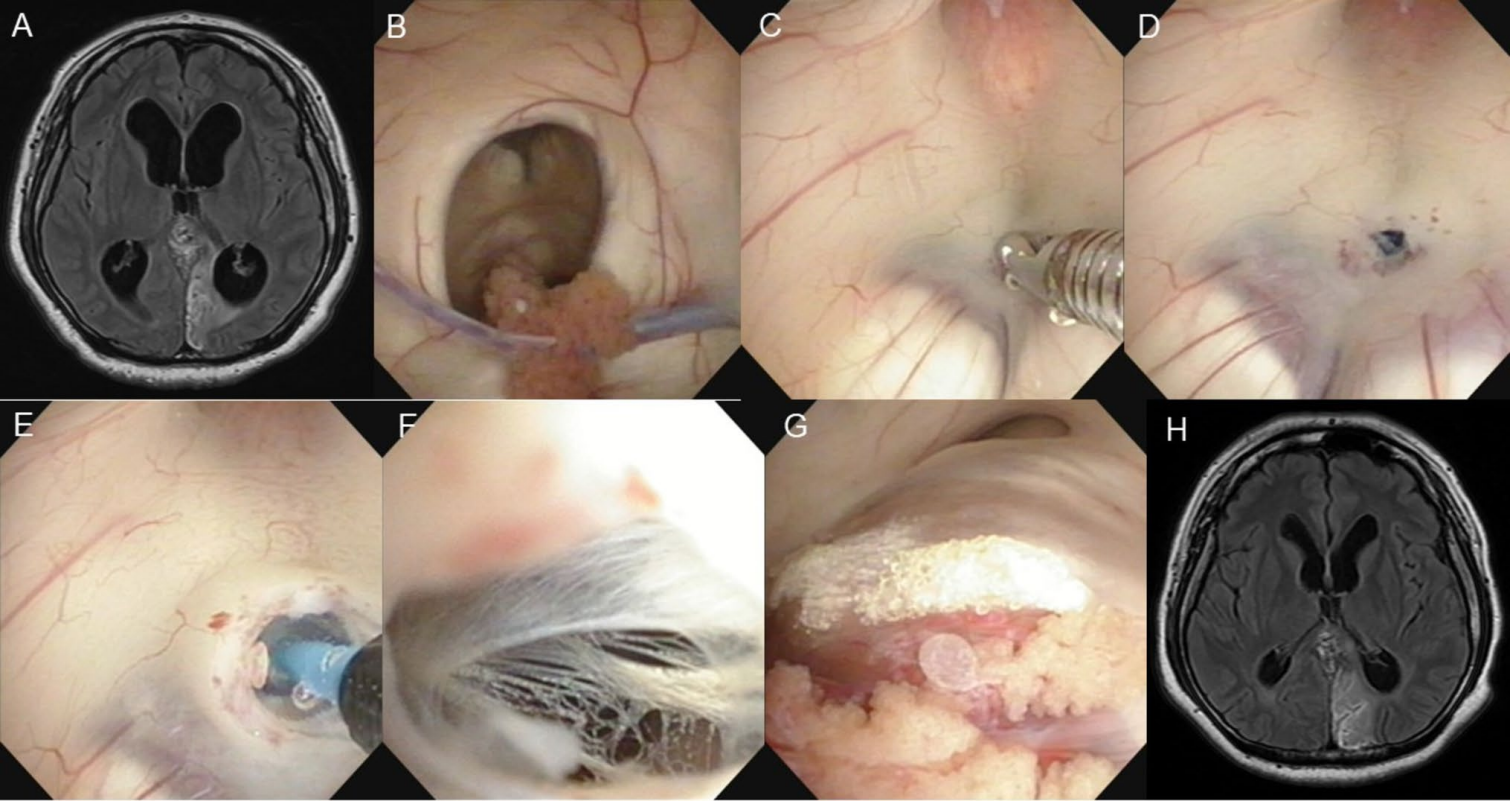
Four days after the second TAE treatment of the dural AVF, disturbance of the consciousness worsened, hydrocephalus was worse on his brain MRI A. Emergent endoscopic third ventriculostomy (ETV) was performed. Right foramen of Monro was dilated B. Puncture was made at the midpoint between the bilateral mammillary bodies and infundibular recess C, D. Puncture hole was dilated with balloon catheter E, making clearly visible to the quadrigeminal cistern F. Aqueductal occlusion due to the varix of the dural AVF was observed G. Post-ETV MRI showed the improvement of the hydrocephalus H
